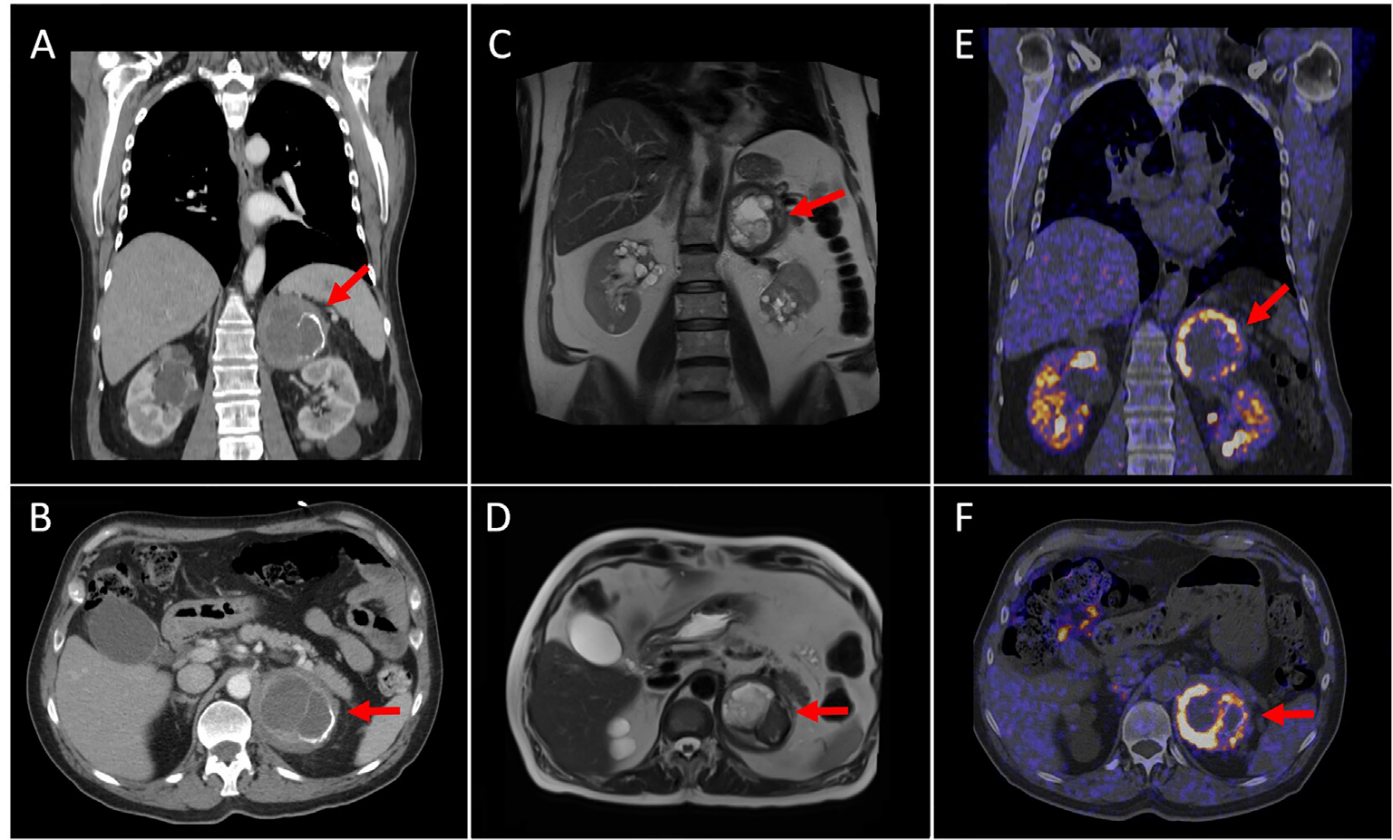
Coronal and axial CT views (A, B) show an 8 cm left adrenal tumor with a cystic component inside and partially calcified wall. Coronal and axial MRI views (C, D) reveal a left adrenal mass with a heterogeneous content (necrotic-cystic areas). Coronal and axial [^18^ F] DOPA PET/CT images (E, F) demonstrate intense and heterogeneous DOPA-uptake in the periphery of the mass (SUVmax of 20.9). These findings were compatible with the existence of pheochromocytoma with a cystic–necrotic–hemorrhagic component.
